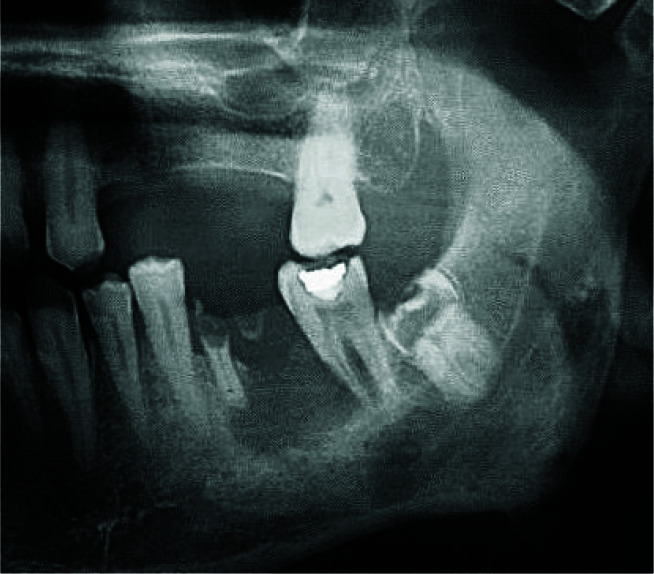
Panoramic radiograph shows an ill-defined radiolucent lesion in area of #18, #19 with bone sclerosis, residue of first molar root and resorption of mesial root of #18
